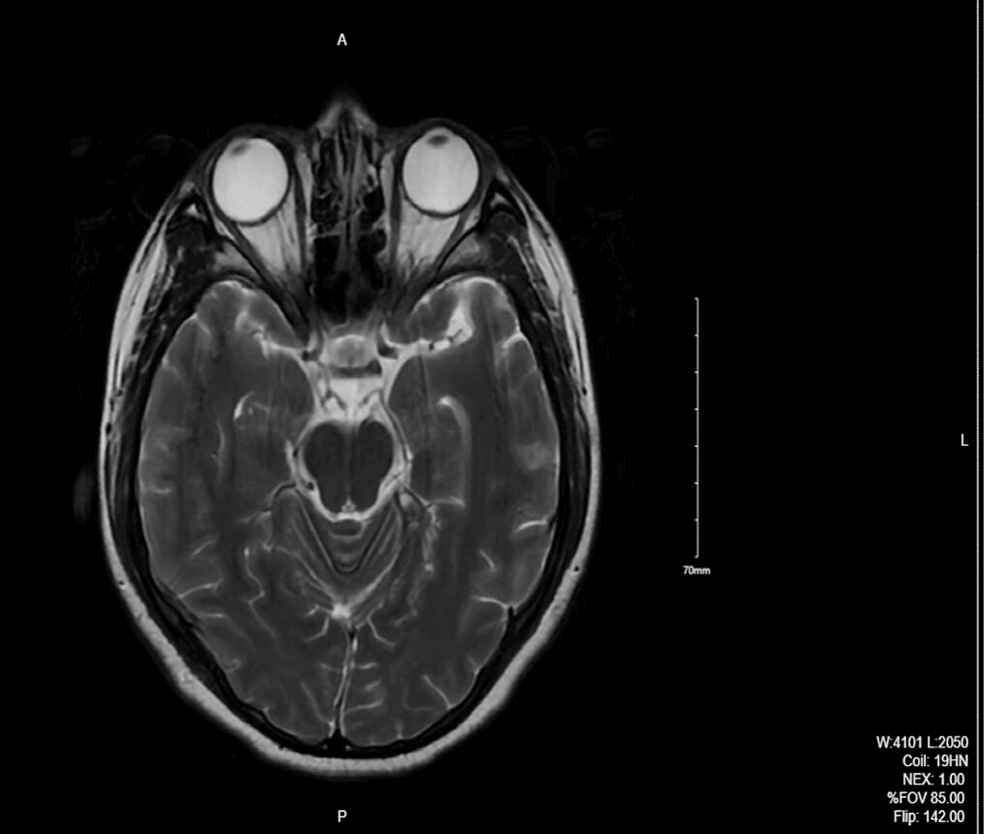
MRI of the orbits with contrast: The globes show mild protrusion of the optic discs and flattening of the posterior sclera bilaterally.
